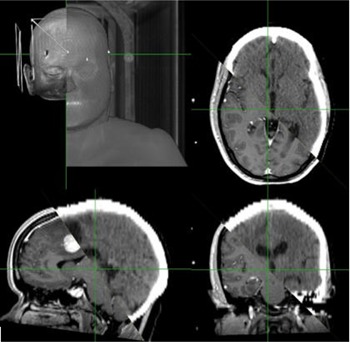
Overall, axial, coronal, and sagittal views (clockwise from top left) of CT/MR fusion in CK MultiPlan.
